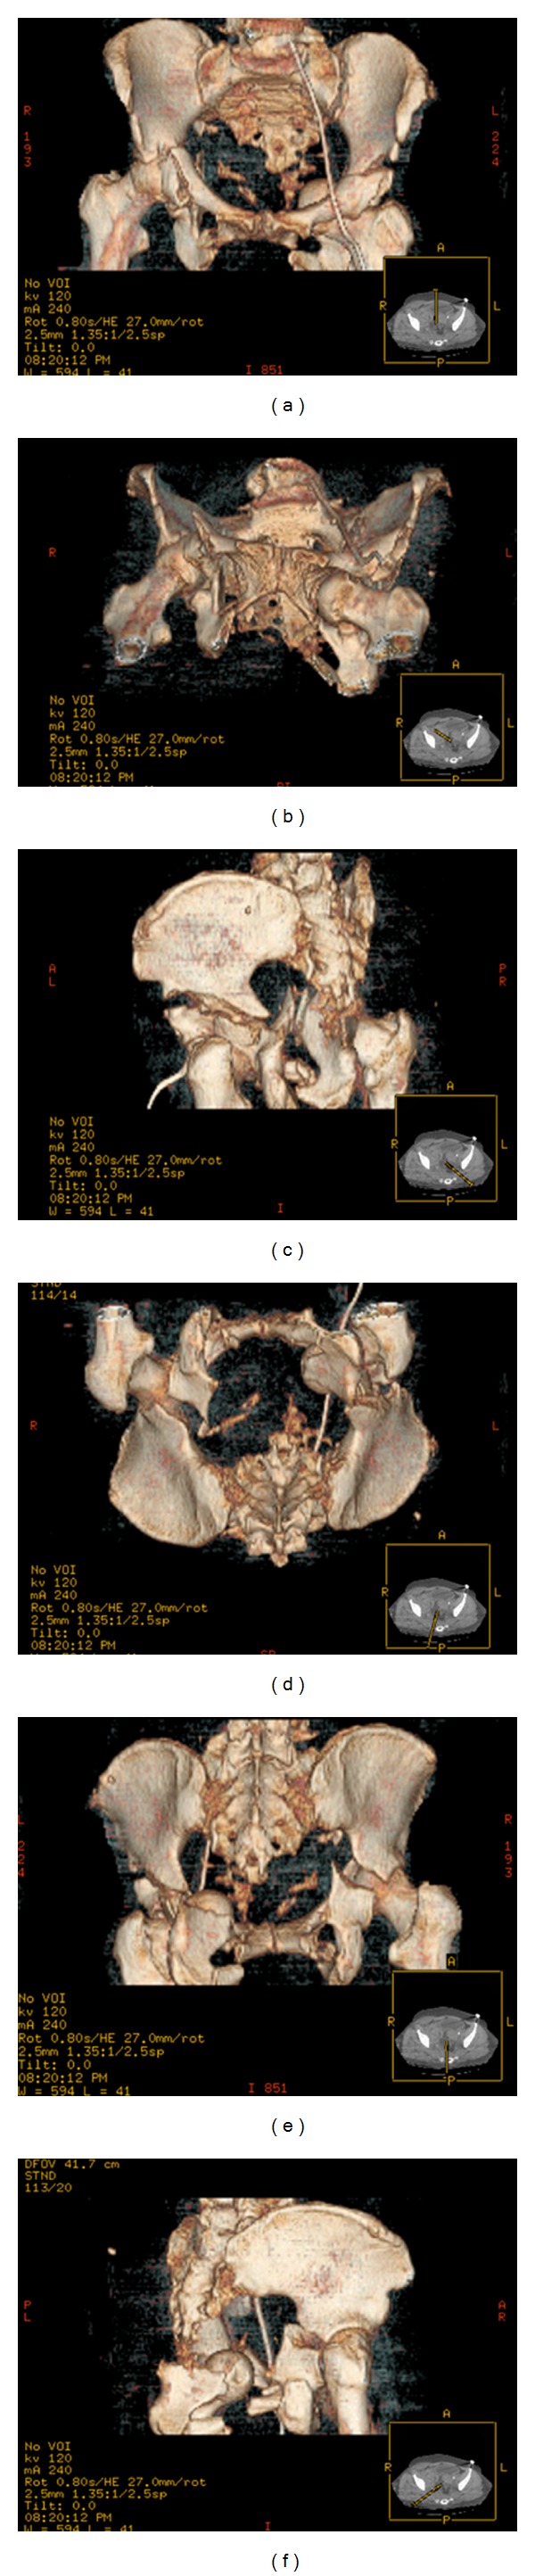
(a) 3D CT reconstruction of pelvis—courtesy of the Botsford General Hospital Radiology Department (anterior-posterior view). (b) 3D CT reconstruction of pelvis—courtesy of the Botsford General Hospital Radiology Department (anterior-inferior view). (c) 3D CT reconstruction of pelvis—courtesy of the Botsford General Hospital Radiology Department (lateral: left to right view). (d) 3D CT reconstruction of pelvis—courtesy of the Botsford General Hospital Radiology Department (inferior view). (e) 3D CT reconstruction of pelvis—courtesy of the Botsford General Hospital Radiology Department (posterior-anterior view). (f) 3D CT reconstruction of pelvis—courtesy of the Botsford General Hospital Radiology Department (lateral: right to left view).
